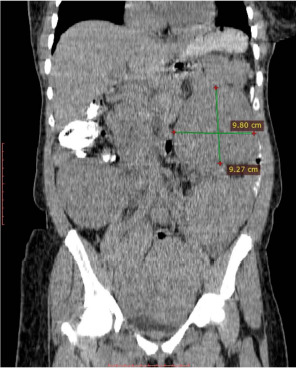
Coronal reformatted abdominal CT scan Image showing multiple isodense round and oval-shaped lesions in the peritoneal cavity before GNRH therapy
